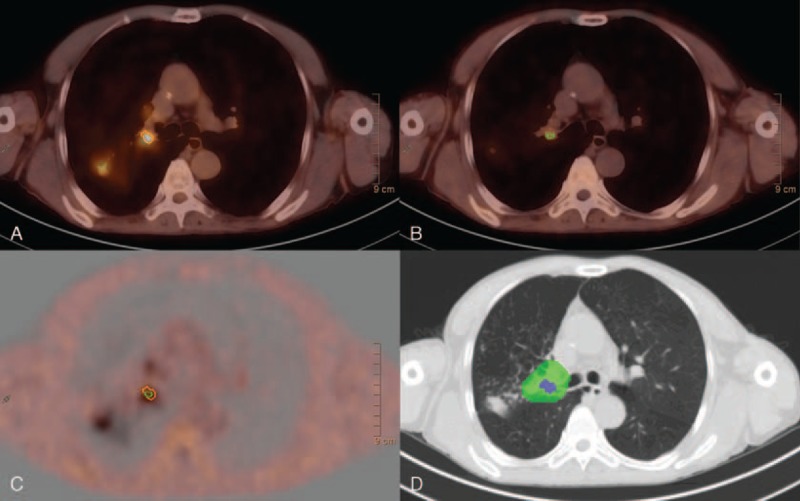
Representative (A) FDG PET/CT, (B) FLT PET/CT, (C) fused PET/CT images, and (D) delineation of target regions of typical primary disease of case 4. The orange lines and the green lines indicate the high-uptake area with threshold of 50% of FDG-SUV_max_ and 80% of FLT-SUV_max_. The green hatched region indicated conventional planning target volume whereas the purple hatched region indicated biological subvolume for boost. CT = computed tomography, FDG = fluorodeoxyglucose, FLT = fluorodeoxythymidine, PET = positron emission tomography, SUV_max_ = maximum standardized uptake value.
